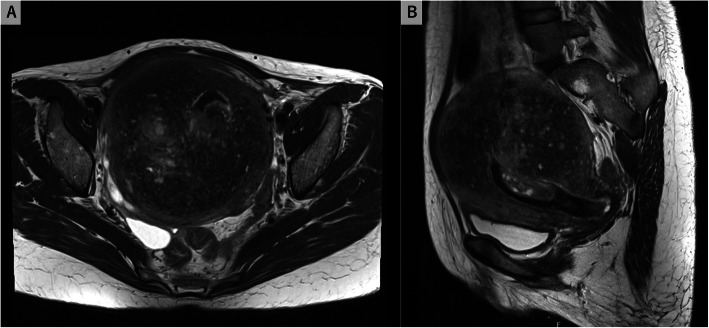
Contrast-enhanced magnetic resonance imaging (MRI) of the pelvis. Despite a high clinical suspicion of *H. influenzae* infection in the uterus, T2-weighted MRI revealed no evidence of uterine abscess formation. The uterus was enlarged due to a known adenomyoma. a horizontal view; b sagittal view
